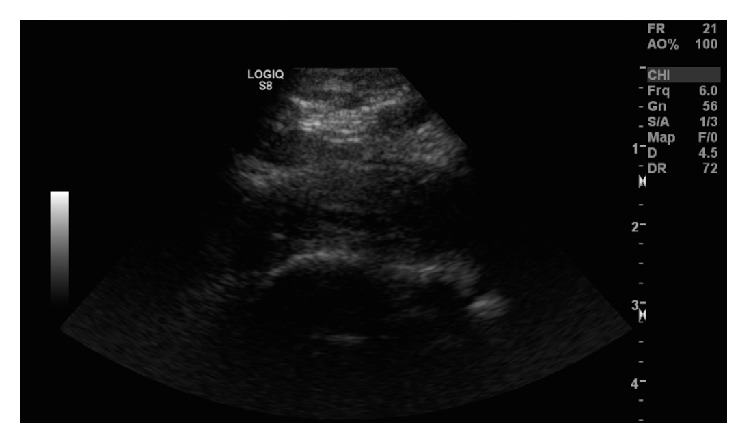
Right upper extremity ultrasound of a 25-year-old barista revealing acute thrombosis of the right central subclavian and axillary veins with compression by the first rib.
